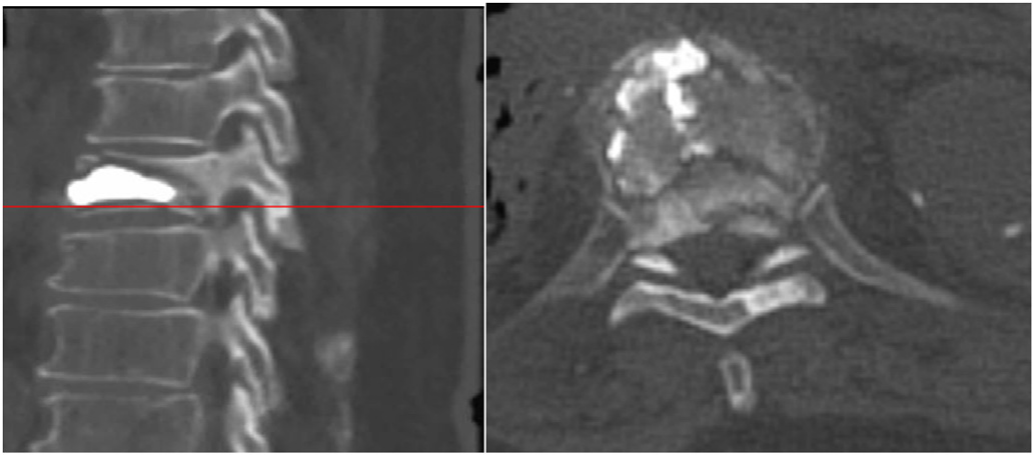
CT without contrast T8 vertebral body fracture with retropulsion of bone fragments contributing to moderate to severe canal stenosis.
